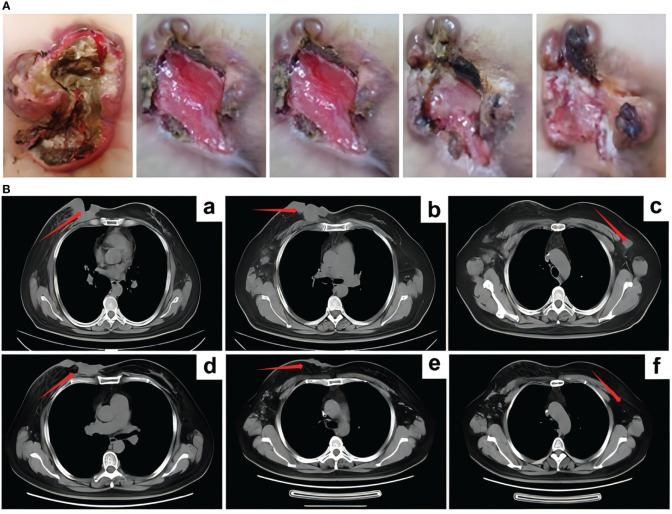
The curative effect of RC48 treatment. (A) After RC48 treatment, the tumor rupture was smaller than before. (B) CT efficacy evaluation after 6 cycles of RC48 treatment. (a-c) Patient status before RC48 treatment; (d-f) after RC48 treatment, the breast tumor and bilateral axillary lymph nodes were significantly reduced.
